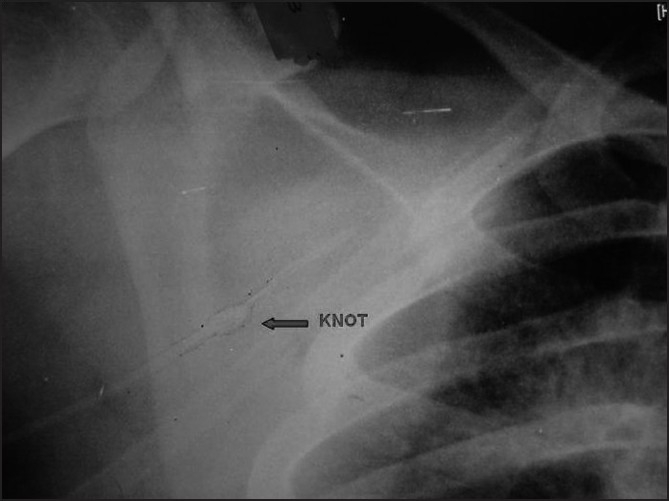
Magnified view of the immediate postoperative chest X-ray suggestive of a knot in the catheter identified by retrospective scrutiny
